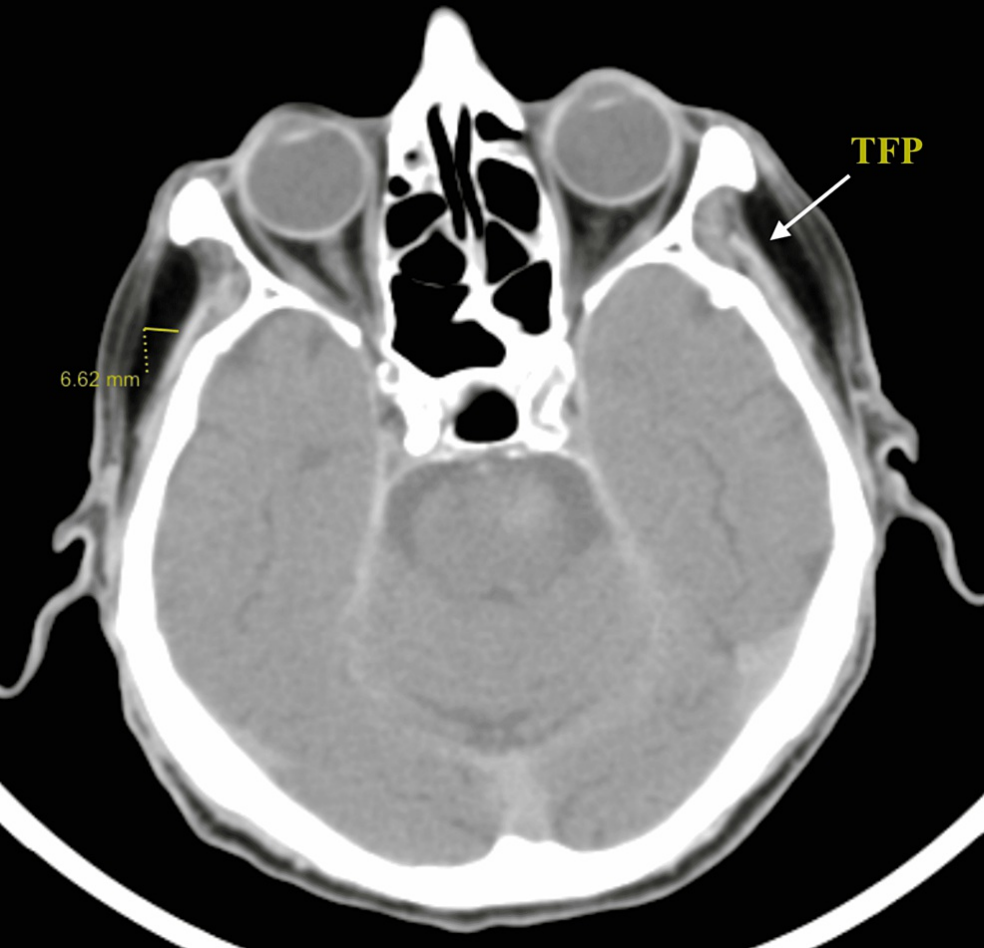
The patient’s head CT scan demonstrating a cross-sectional measurement of the temporal fat pad (TFP).
